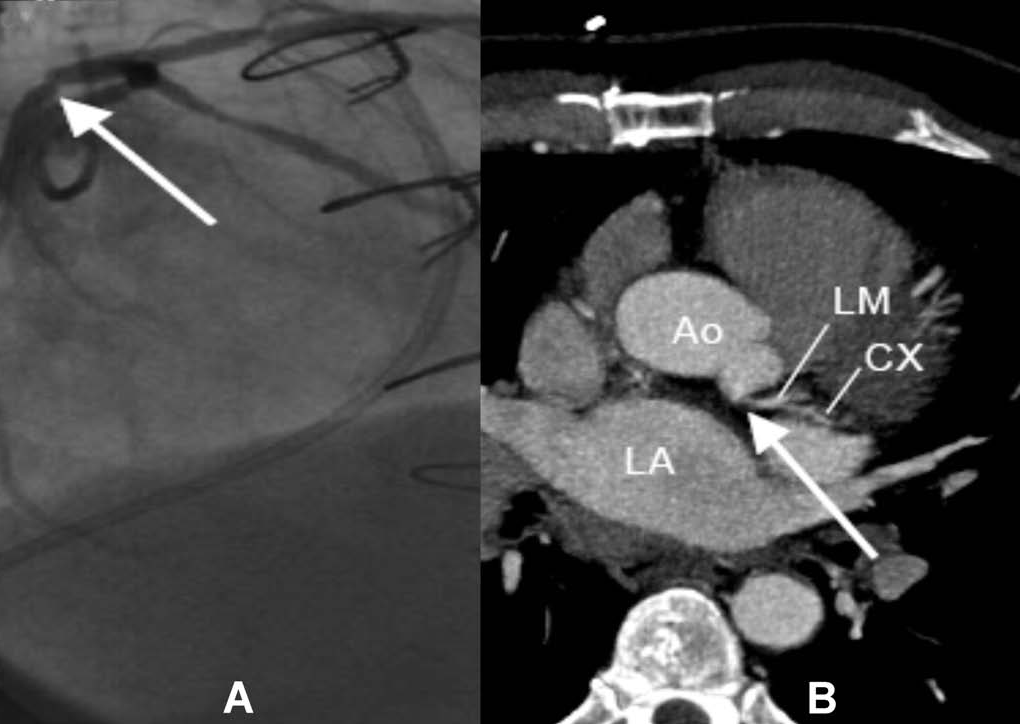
A: Coronary angiogram showing the stenosis of the left main stem in patient number 2 (arrow, LAO: left anterior oblique). B: Multi-slice computed tomography of the same patient with the corresponding stenosis (arrow, MIP: maximal intensity projection). Ao: aorta, LA: left atrium, LM: left main artery, CX: circumflex artery.
